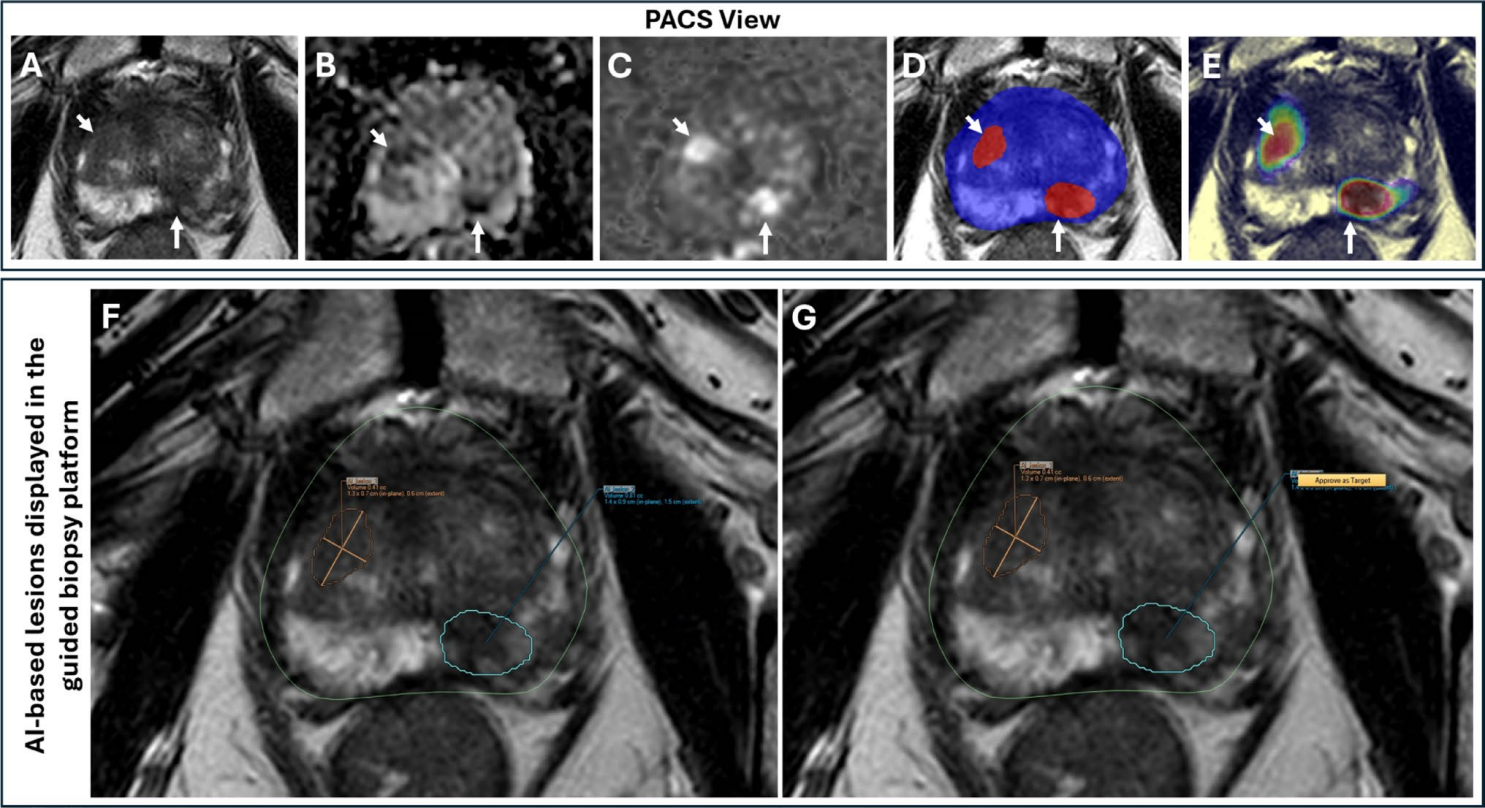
Biopsy planning system integration. 76-year-old patient with a serum PSA of 8.4 ng/ml. Bi-parametric MRI (A = T2W MRI, B = ADC map, C = b1500 DW MRI) demonstrates focal lesions in the left apical peripheral zone (arrow) and right apical anterior transition zone (short arrow). Binary lesion prediction (D) and probability (E) maps confirm presence of both lesions (long and short arrows). AI-model outputs are presented to users in the biopsy preparation platform (F) and the platform allows user to approve or reject the AI-based target lesions (G)
